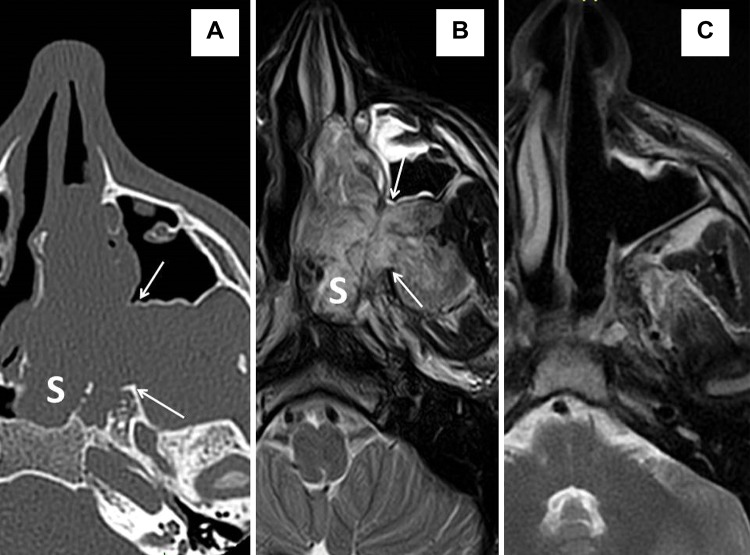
Juvenile nasopharyngeal angiofibroma, case P5, Radkowski grade 2c. Axial CT (A) and MRI T2-weighted scans (B) showing a mass in the dorsal two thirds of the left nasal cavity and in the sphenoid sinus *(marked by S)* with a bulky extension to the pterygopalatine fossa and the ITF. Extensive anteroposterior widening of the sphenopalatine foramen is marked by *arrows*. (C) An axial MRI T2-weighted scan one year after surgery demonstrating no evidence of disease.
